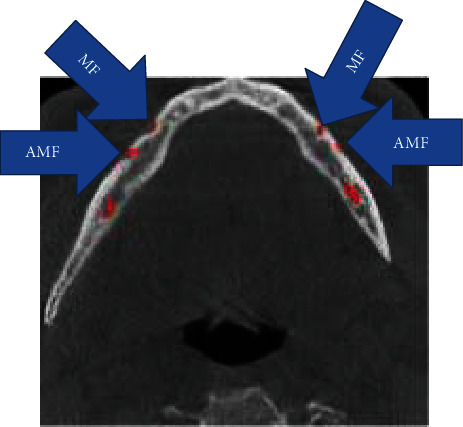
Right and left AMF at axial CBCT.
